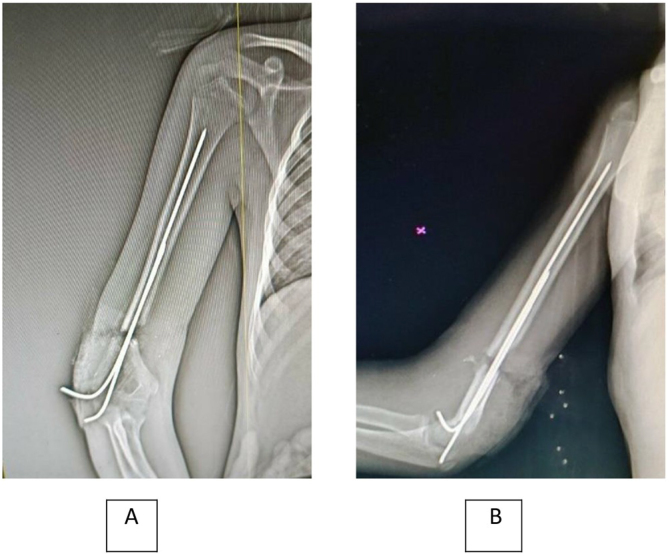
(A) Post-operative anterior X-ray. (B) Post-operative posterior X-ray; showing the internal fixation by k. wires.
